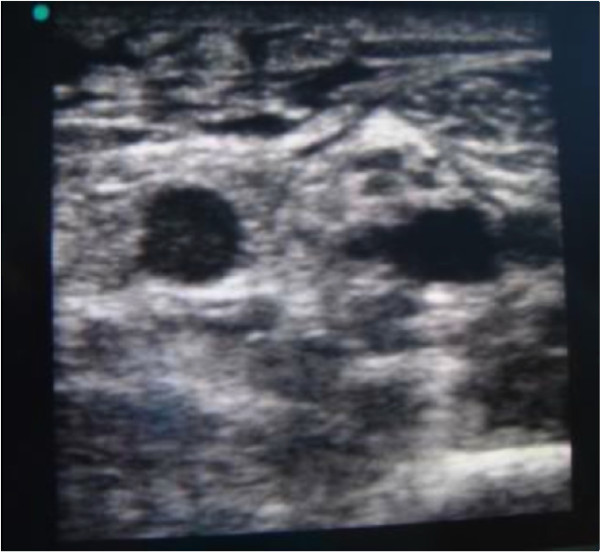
Ultrasound scan at the mid-arm. Left to right: basilic vein, brachial nerve, and brachial artery between two small brachial veins.
